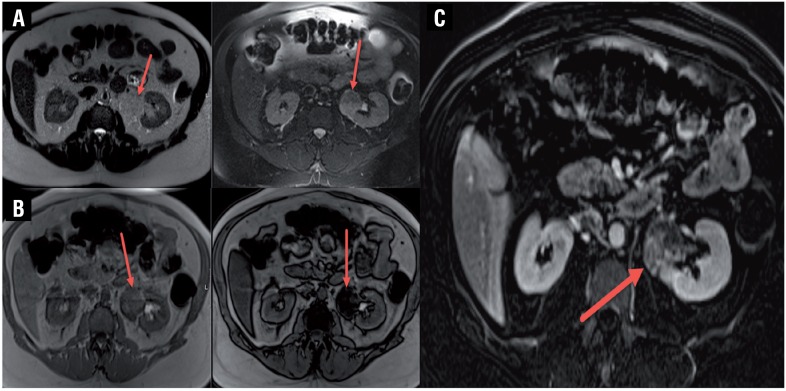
MRI Images. a) Axial MRI, HASTE (T2-weighted) (left) and HASTE with fat-saturation (right) demonstrates the mass. b) Axial MRI, in phase (left) and opposed phase (right), c) Axial MRI, post-contrast subtracted image shows areas of enhancement in mass.
